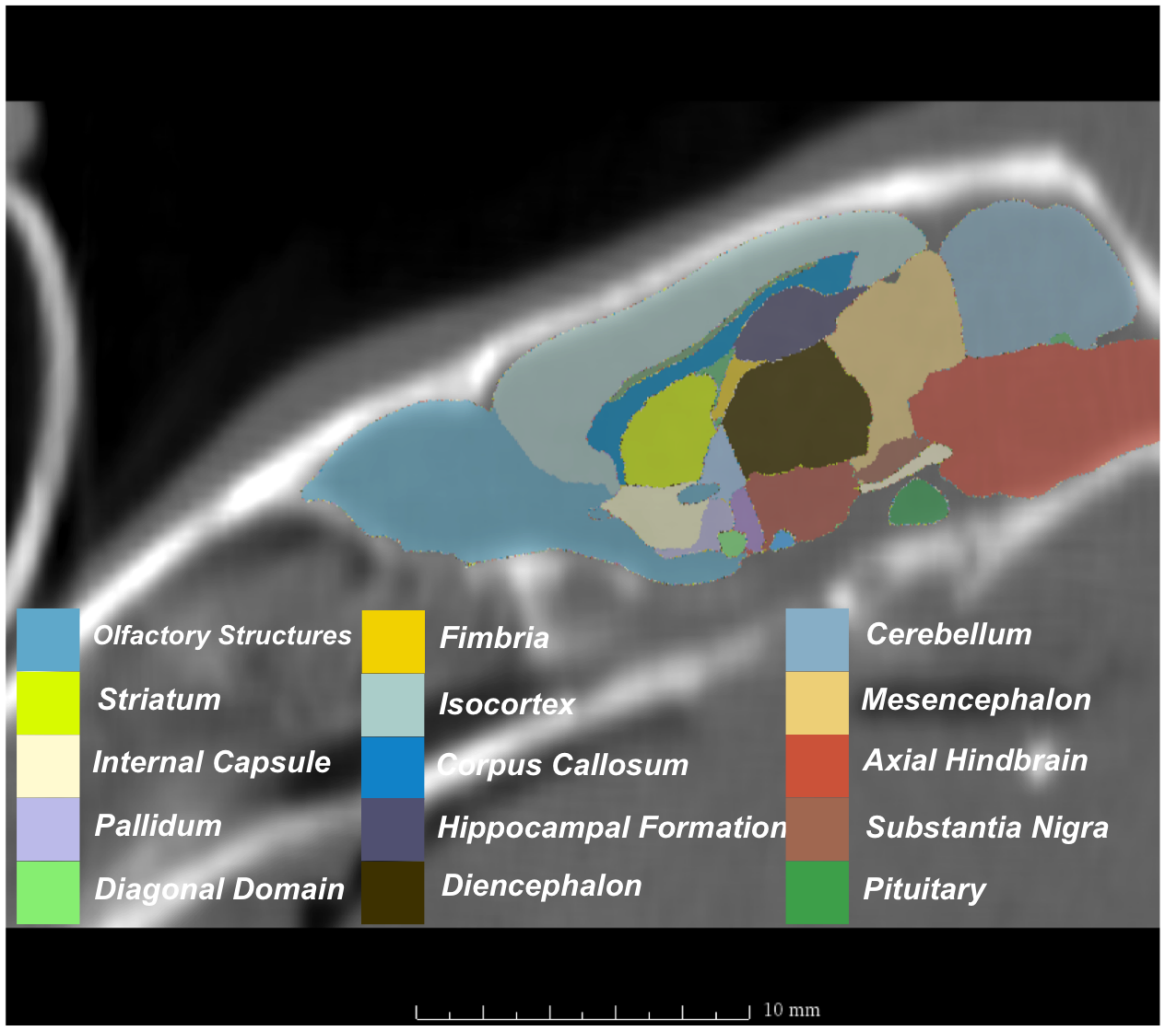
MRI with labeled structures from atlas registered to the CBCT.
